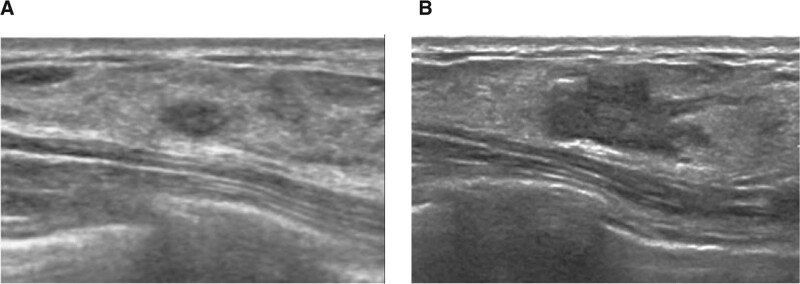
Screening US of 32-yr old woman with a family history of breast cancer. (A) Prior US showed 0.6 cm sized oval and circumscribed hypoechoic mass. The mass was categorized as BI-RADS category 3 in both initial and reassessment. (B) On follow-up US after 14 mo, the mass had increased to 1.9 cm with a morphologic change to irregular shape and angular margin. This mass was confirmed as ductal carcinoma in situ. BI-RADS = Breast Imaging Reporting and Data System, US = ultrasound.
